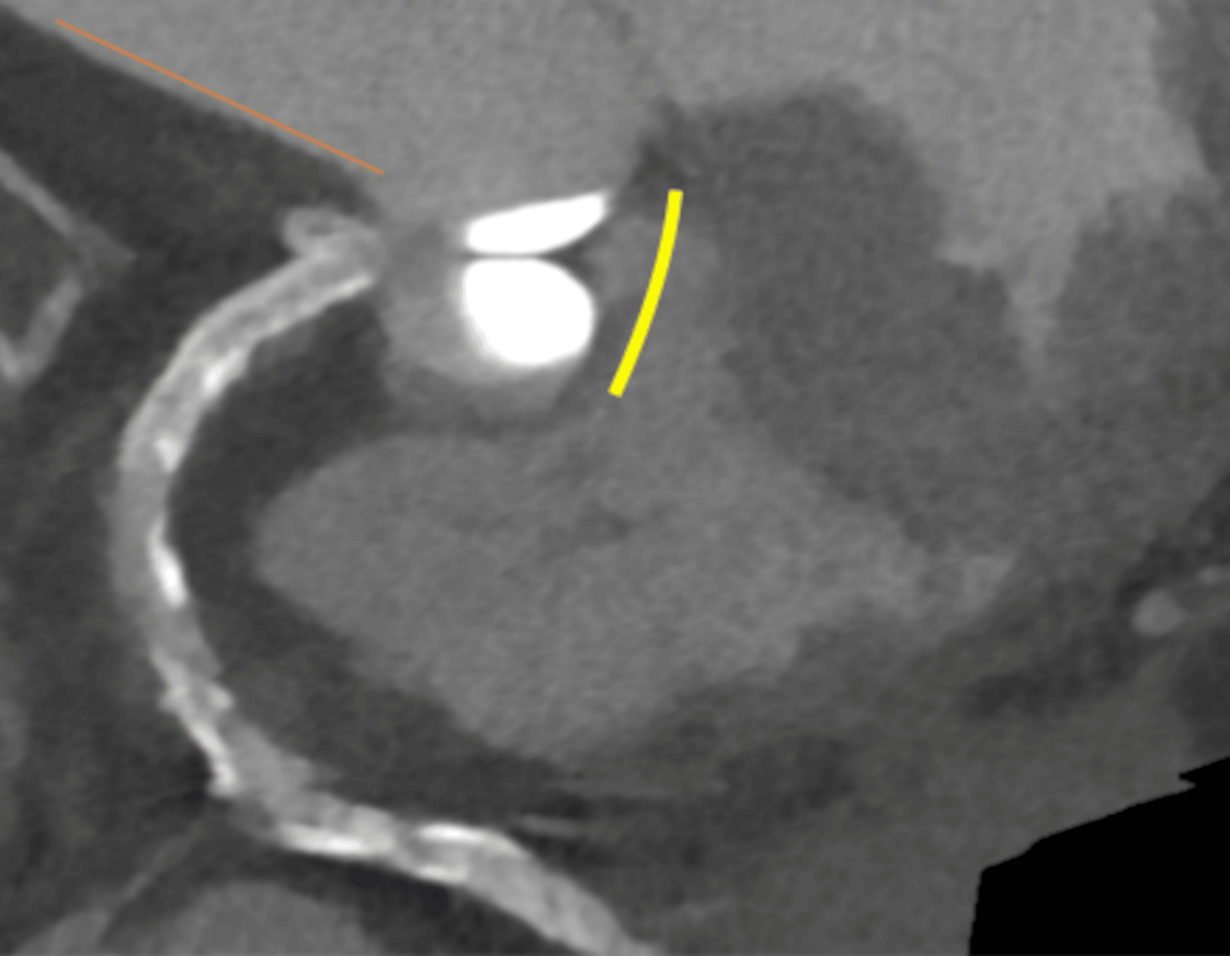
Postprocedural contrast-enhanced computed tomography. Axial contrast-enhanced CT imaging demonstrates retained contrast within the sinus of Valsalva (yellow line) without evidence of ascending aortic dissection (orange line).
